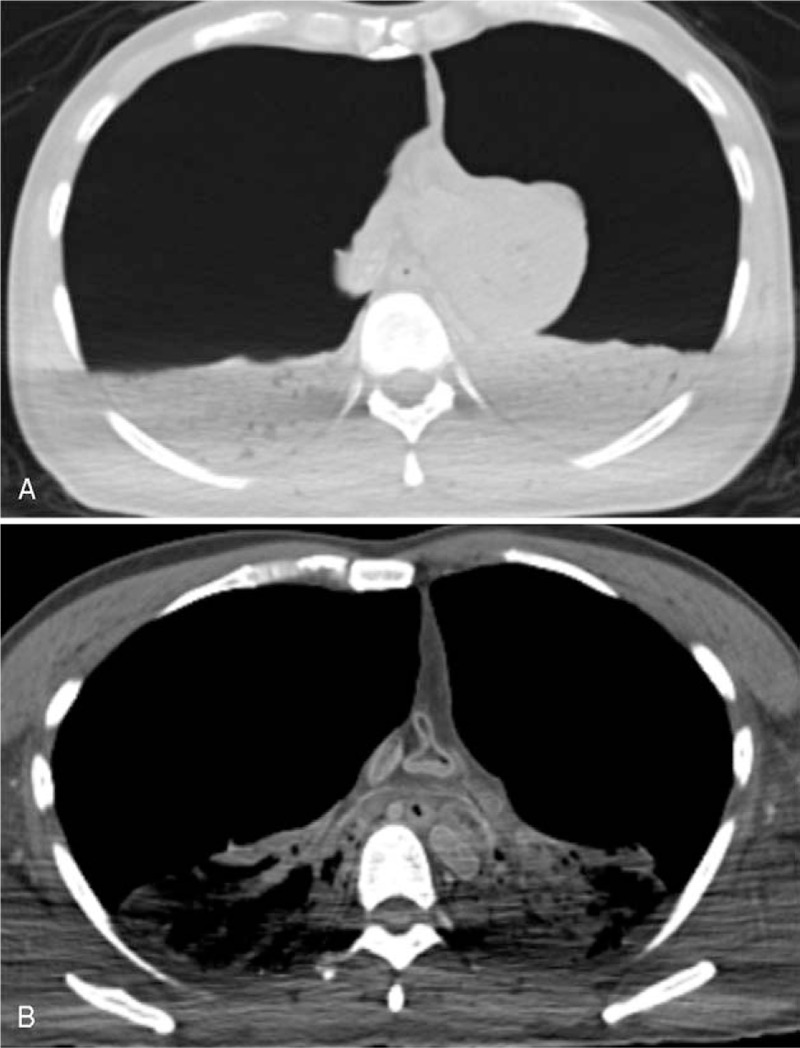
PMCT findings in (A) the lung window, (B) the mediastinal window. Both lungs were collapsed and the density of both lungs was significantly elevated. Mediastinal compression and compression of heart and major vessels were also detected. PMCT = postmortem computed tomography.
